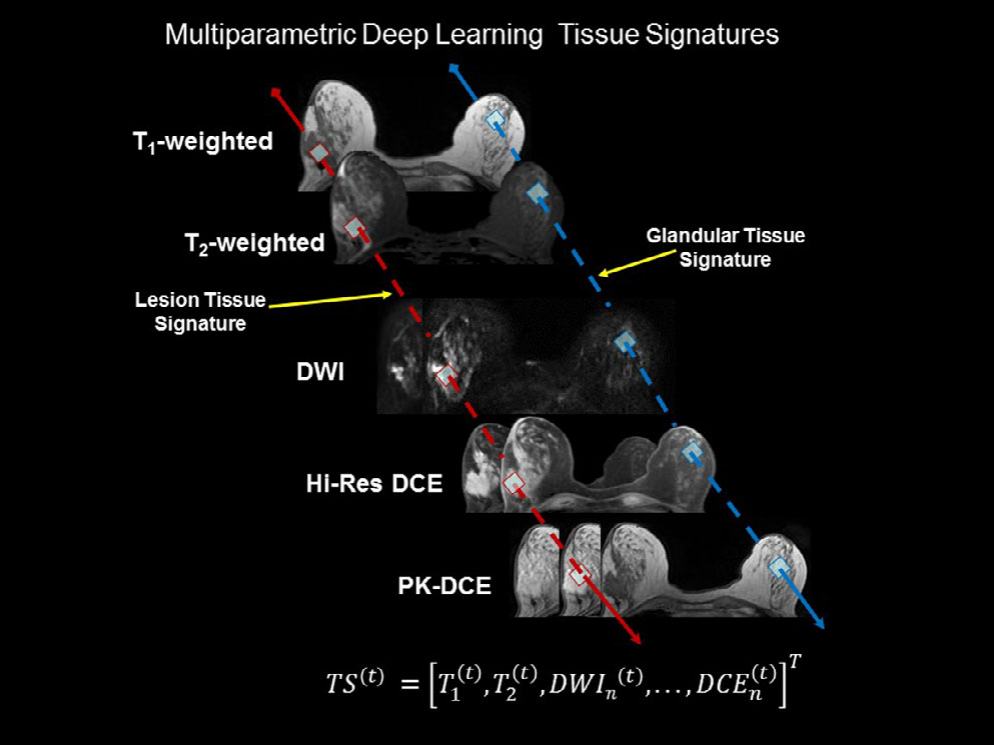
Demonstration of the tissue signatures (TS) defined on multiparametric breast MRI. Representative tissue signatures are shown for normal (glandular‐blue) and lesion tissue (red) from each input MRI. We define the tissue signature as a vector, TS^(ι)^, where (*ι*) (τ) defines the tissue type over each MRI image and n is the number of images each of respective sequence. There were T_1_‐weighted, T_2_‐weighted, high‐resolution (Hi‐Res) and pharmacokinetic (PK) dynamic contrast‐enhanced (DCE) images along with Diffusion Weighted Images (DWI) in the data set. [Color figure can be viewed at http://www.wileyonlinelibrary.com]
